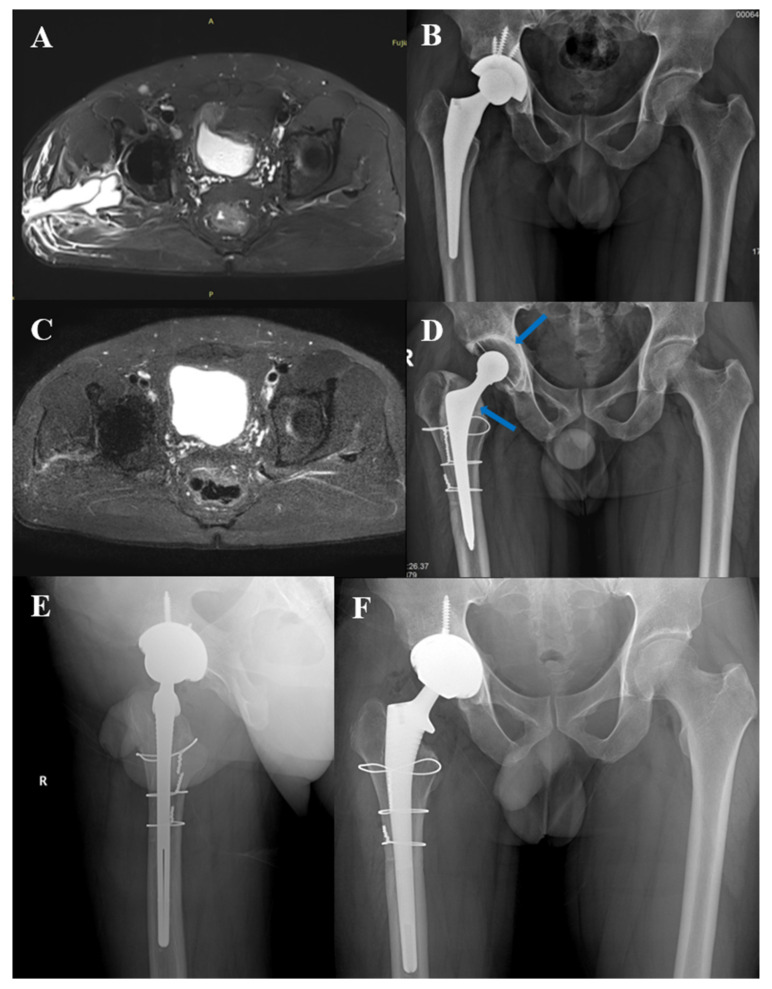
Typical case 1. Male, 41 years old, right total hip arthroplasty. (A): MARS MRI before stage I resection surgery; (B): X-ray before stage I resection surgery; (C): MARS MRI before stage II reimplantation; (D): X-ray before stage II reimplantation, the arrow shows bone cement spacer containing metal; (E,F): X-ray after stage II reimplantation.
